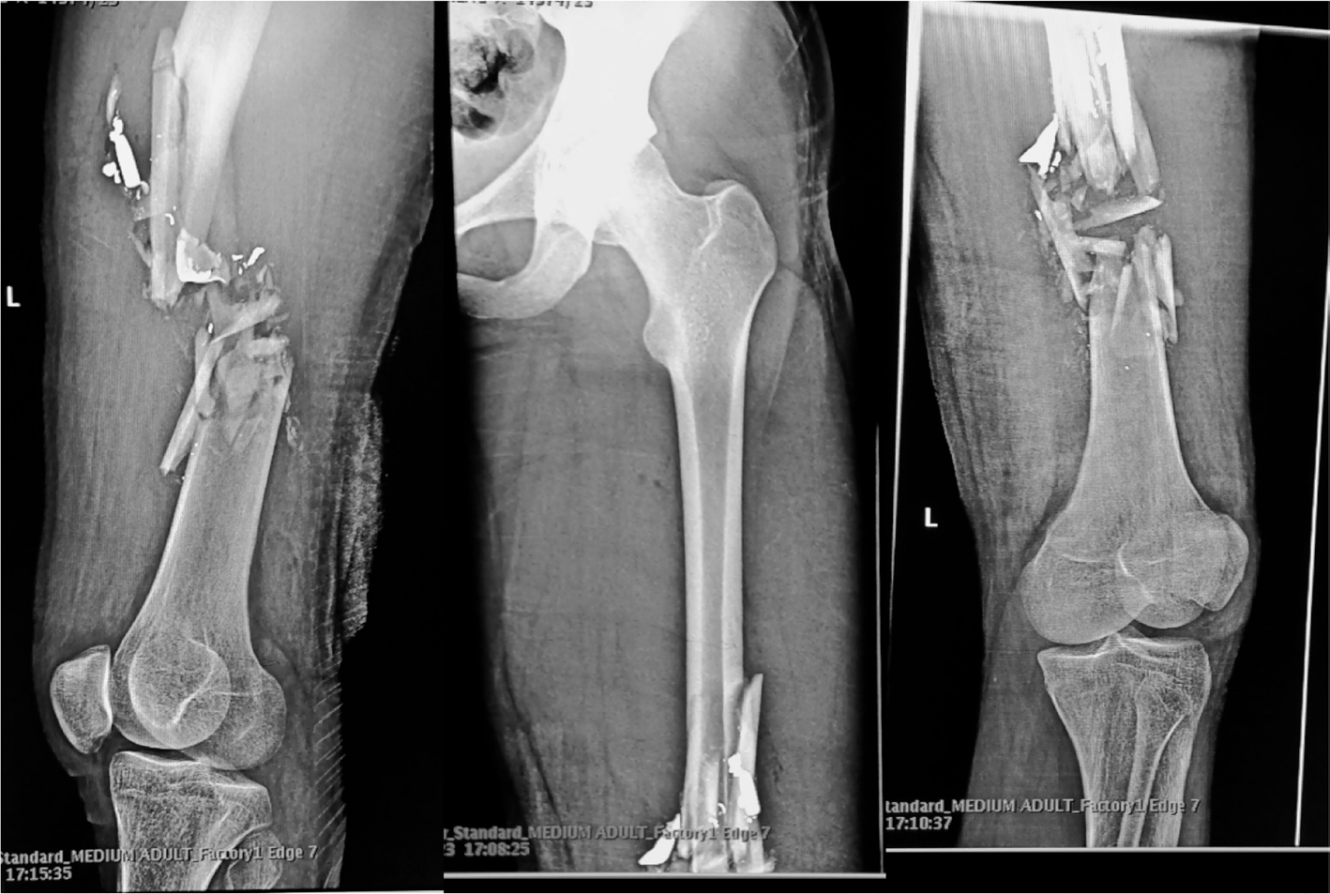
Lateral shoot-through X-rays of the left femur show severe comminution with sharp spicules involving the distal diaphyseal shaft, characteristic of high-energy trauma. Management of this type of unstable fracture typically involves intramedullary locked nailing, with augmentation using PMMA and autologous bone grafts to enhance mechanical stability and support bone regeneration [54]
